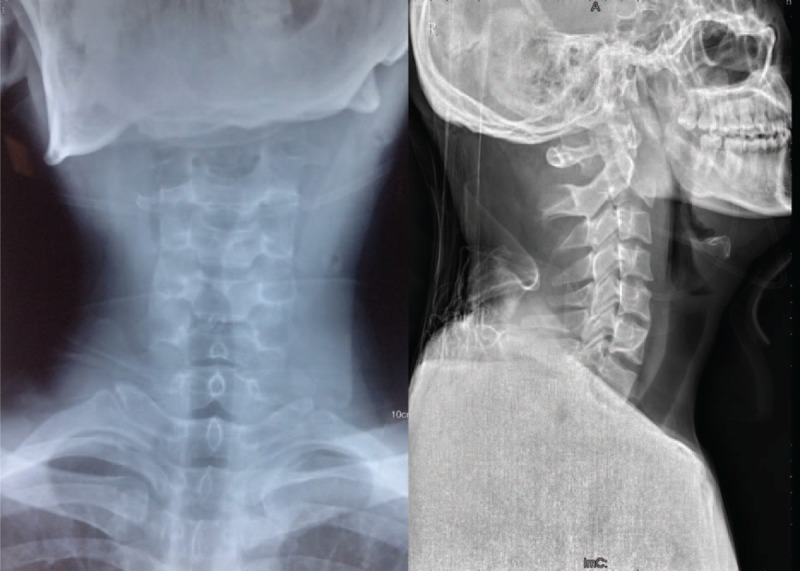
Preoperative anteroposterior and lateral x-rays revealed huge tear drop fracture of anterior–inferior corner of the axis.
